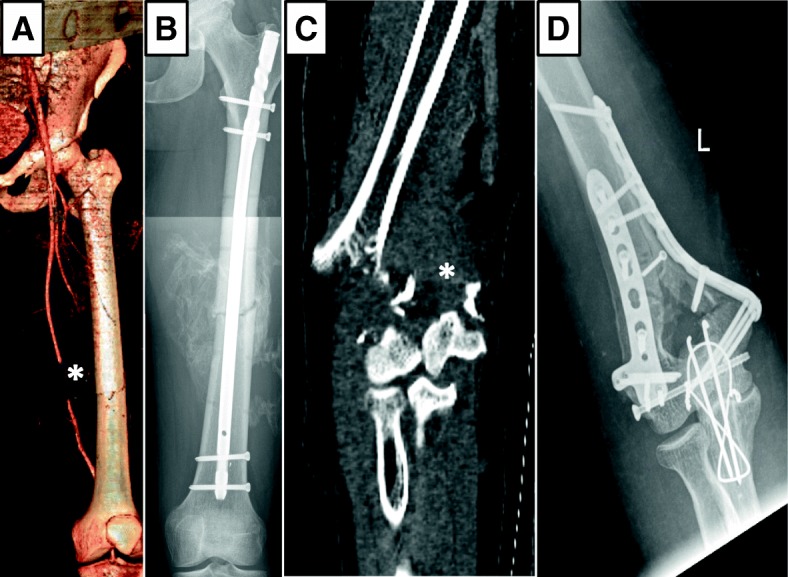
Extremity fractures with associated vascular damage. 3D-reconstruction (a) of the initial CT of a patient after motorcycle accident with closed femoral shaft fracture and disruption of the superficial femoral artery (★) with active bleeding palpable as pulsatile femoral mass (signal loss of soft tissue contrast agent pooling due to 3D-reconstruction sequence). Of note, fracture lines (a) indicate medial translocation of the osseous fragment towards the artery, probably causative of arterial rupture. Initial treatment included immediate open arterial reconstruction with vein graft interposition and external stabilization and after 12 days intramedullary nail repair (b). CTA with active pooling of contrast agent (*) from the brachial artery in a patient after fall with a grade IIIb open fracture of the humeral shaft (c). Initial treatment included patch plasty of the brachial artery, external fixation and fasciotomy of the forearm compartments. After 10 days the elbow joint was reconstructed with open reduction and plate fixation, an olecranon osteotomy was performed for better joint visualization
